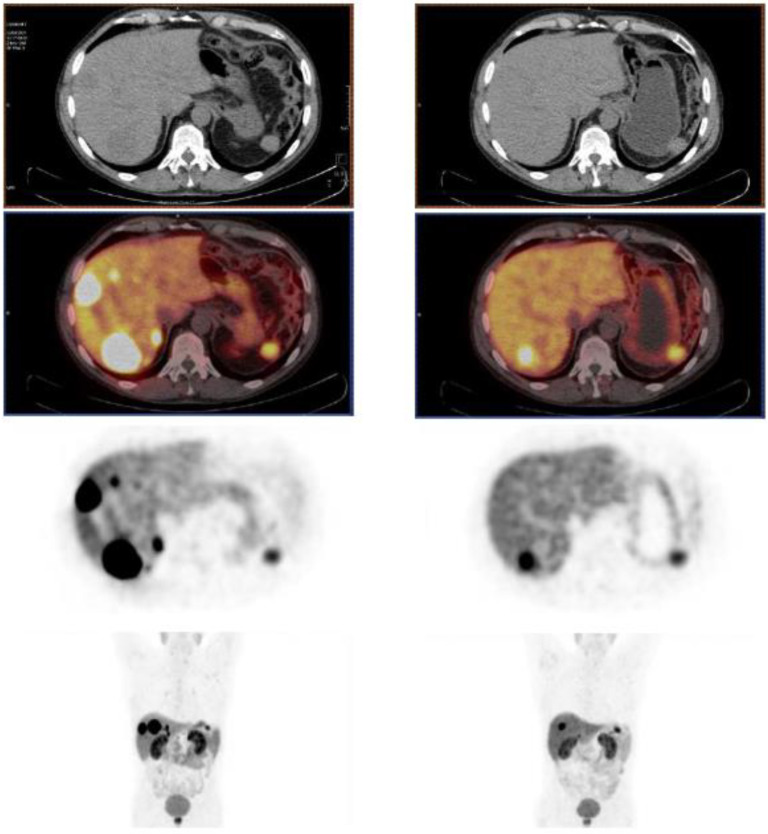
[^68^Ga]Ga–DOTATATE PET/CT in the patient described by Budlewski et al. [227], showing important uptake in hepatic NET metastases after treatment with a long-acting somatostatin analog plus everolimus (left), and significant decrease in lesion uptake after two cycles of [^225^Ac]Ac–DOTATATE associated with good metabolic and structural response (right).
